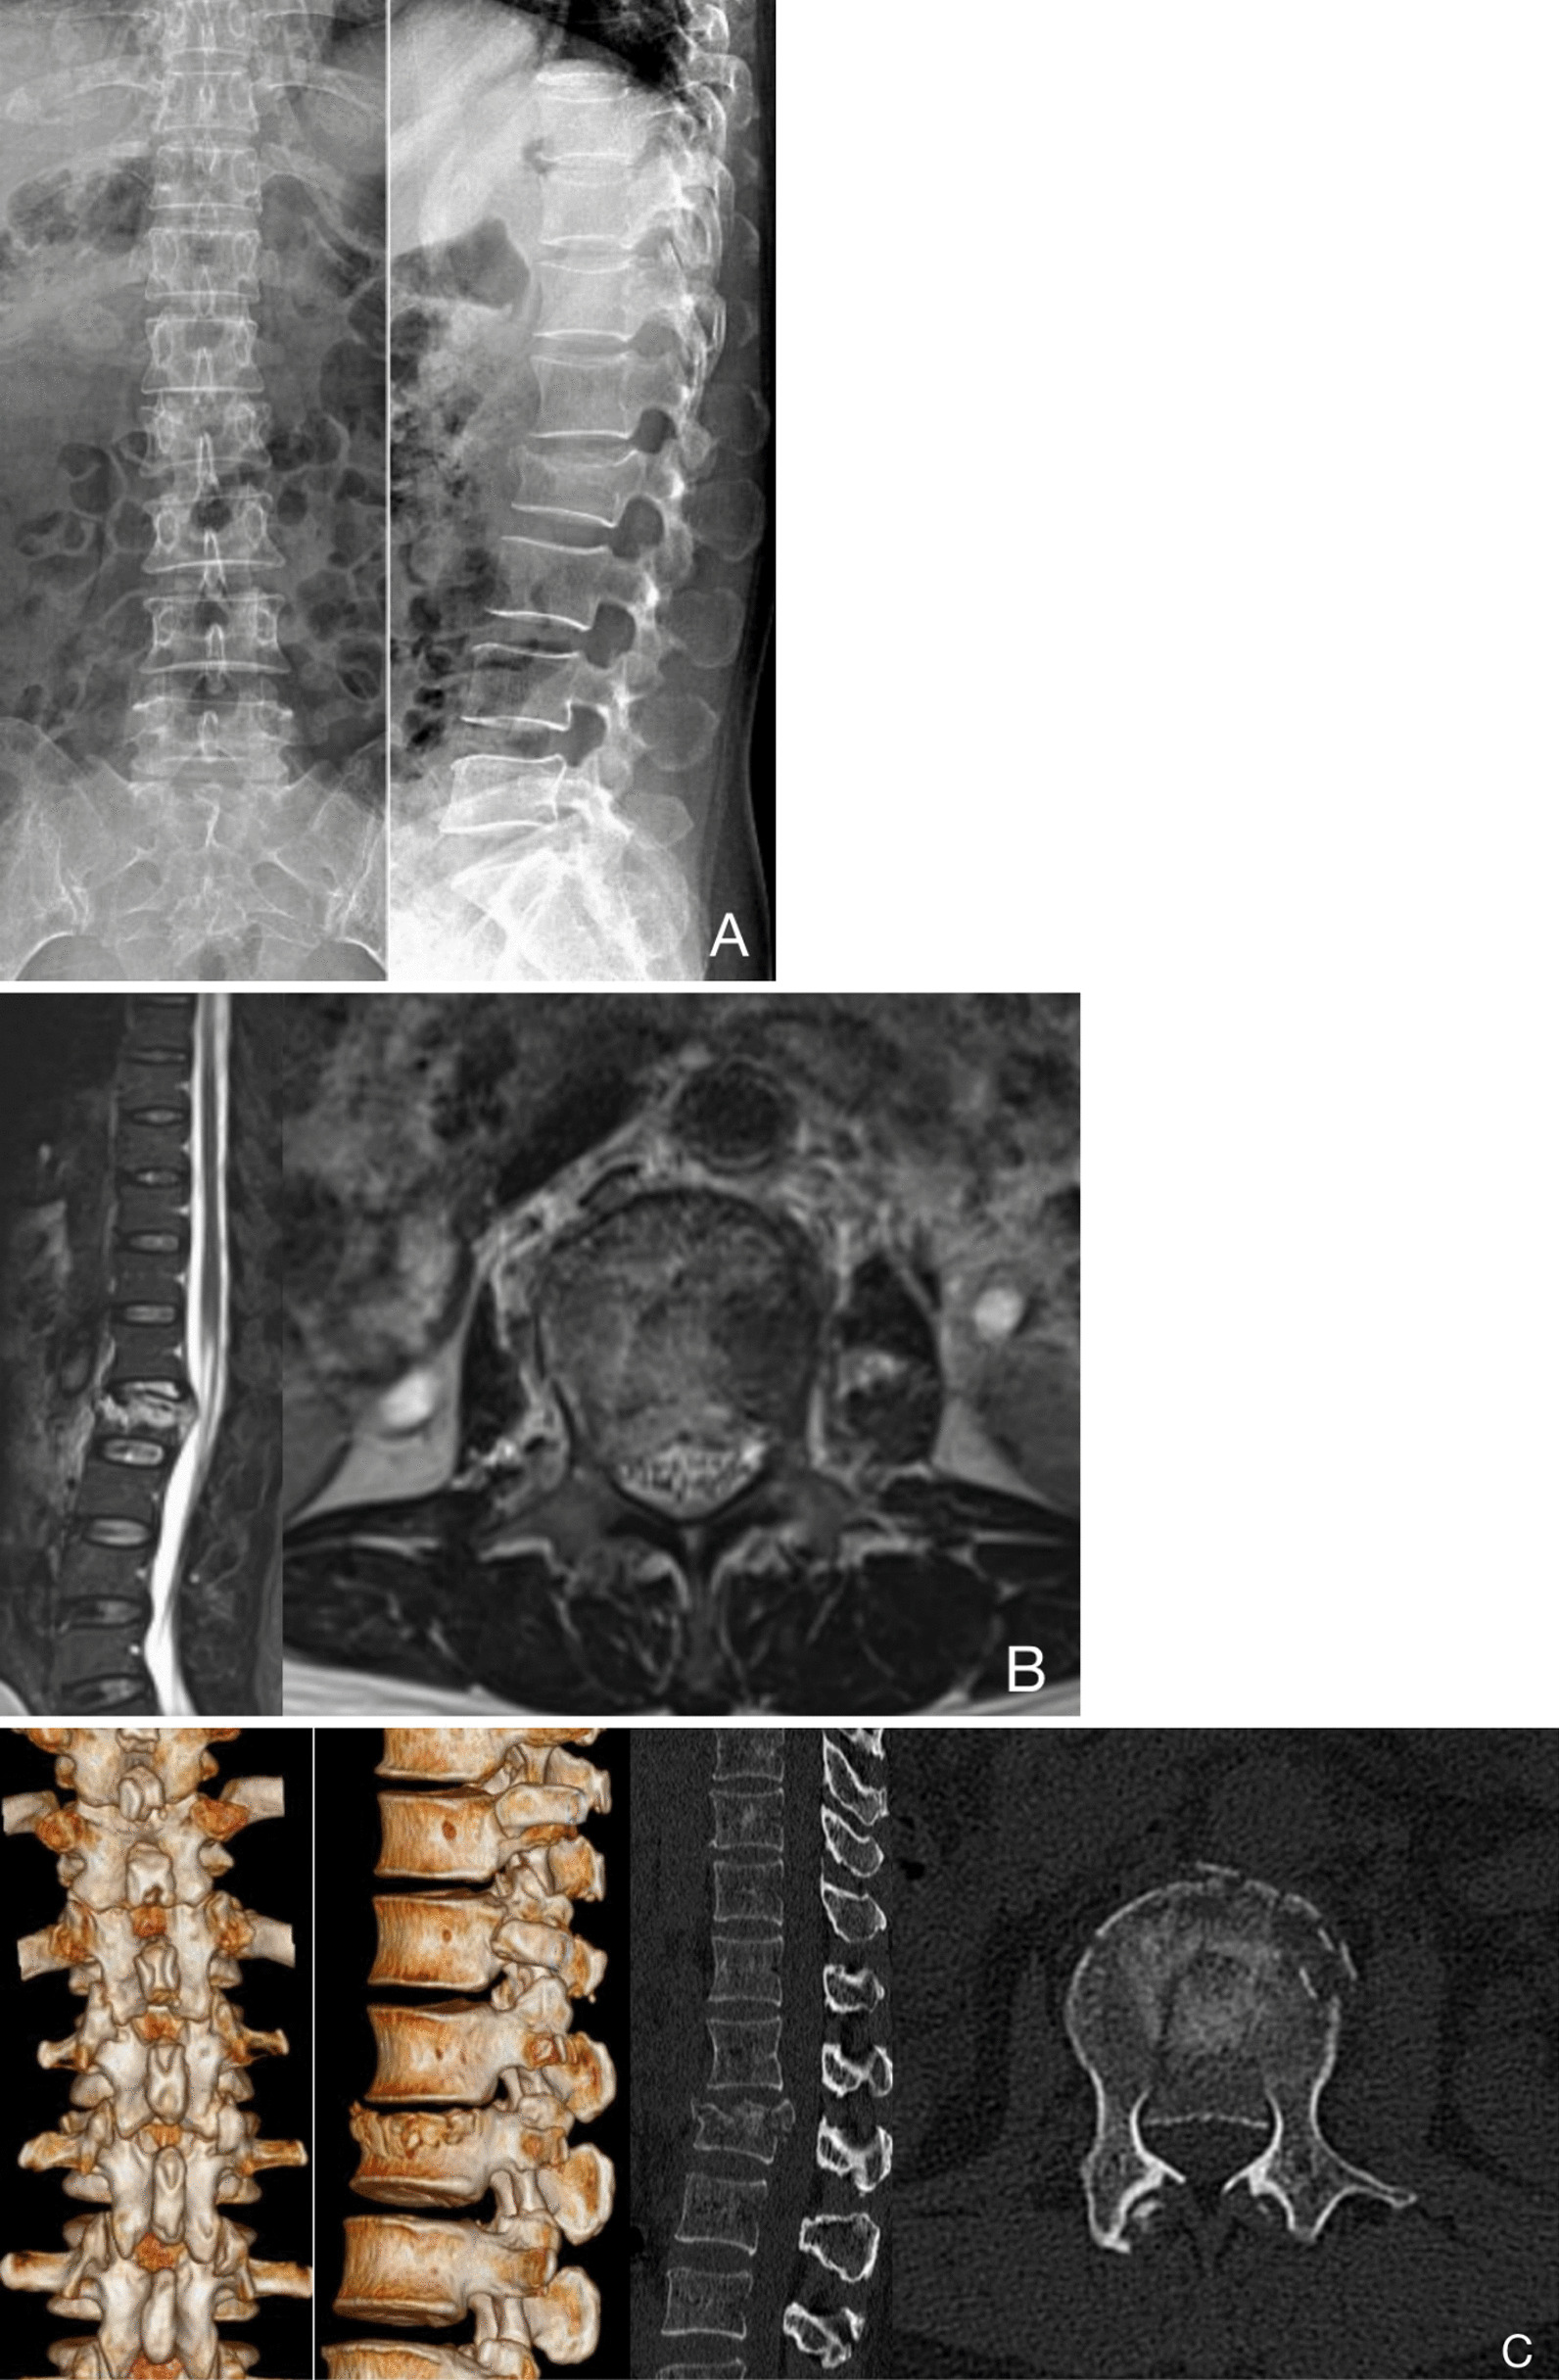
Preoperative images. A Radiograph of a 50-year-old patient who sustained a L2 burst fracture after a car accident. B, C MRI and CT showed that a large bone fragment had herniated into the spinal canal
